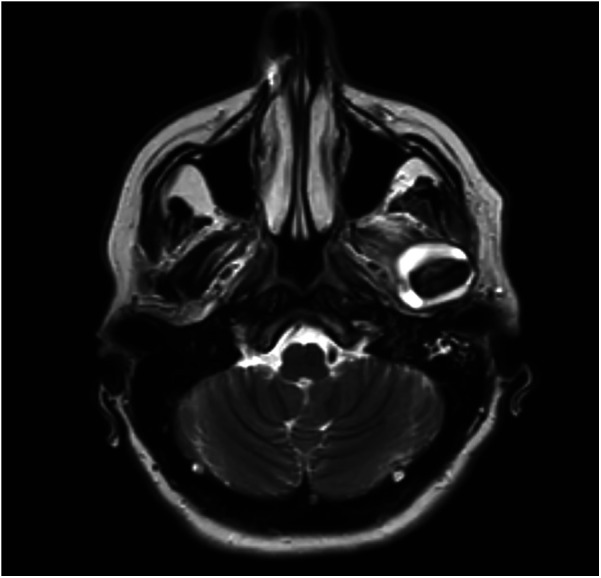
T2 sequence MRI of the TMJ, coronal images, revealing hyperintensity and joint effusion on the left joint.
